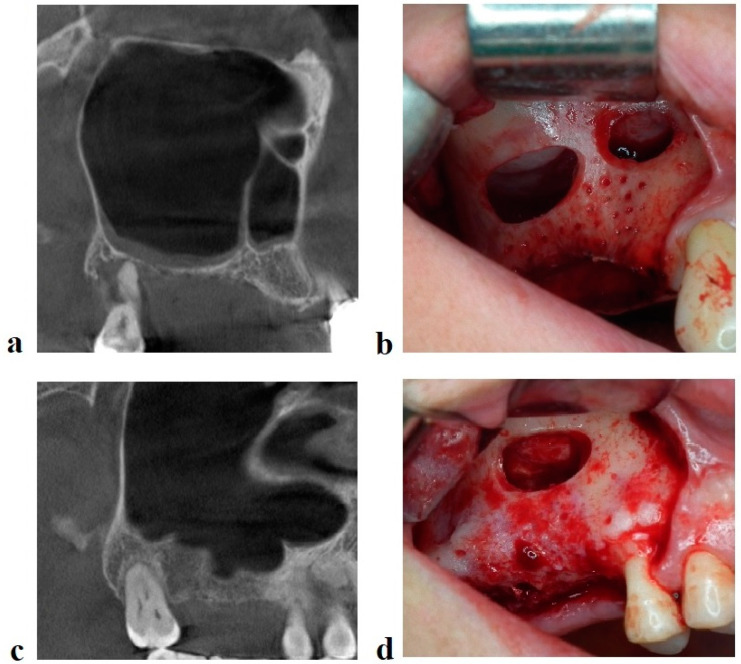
Radiological and clinical aspect of the maxillary sinus in partial edentulous patients before sinus floor augmentation. (a) CBCT sagittal view of a right maxilla with a long medio−lateral oriented septum. (b) Intraoperative view of the two windows technique. (c) CBCT sagittal view of a right maxilla with short medio−lateral oriented septa. (d) Intraoperative view of the osteotomy window.
